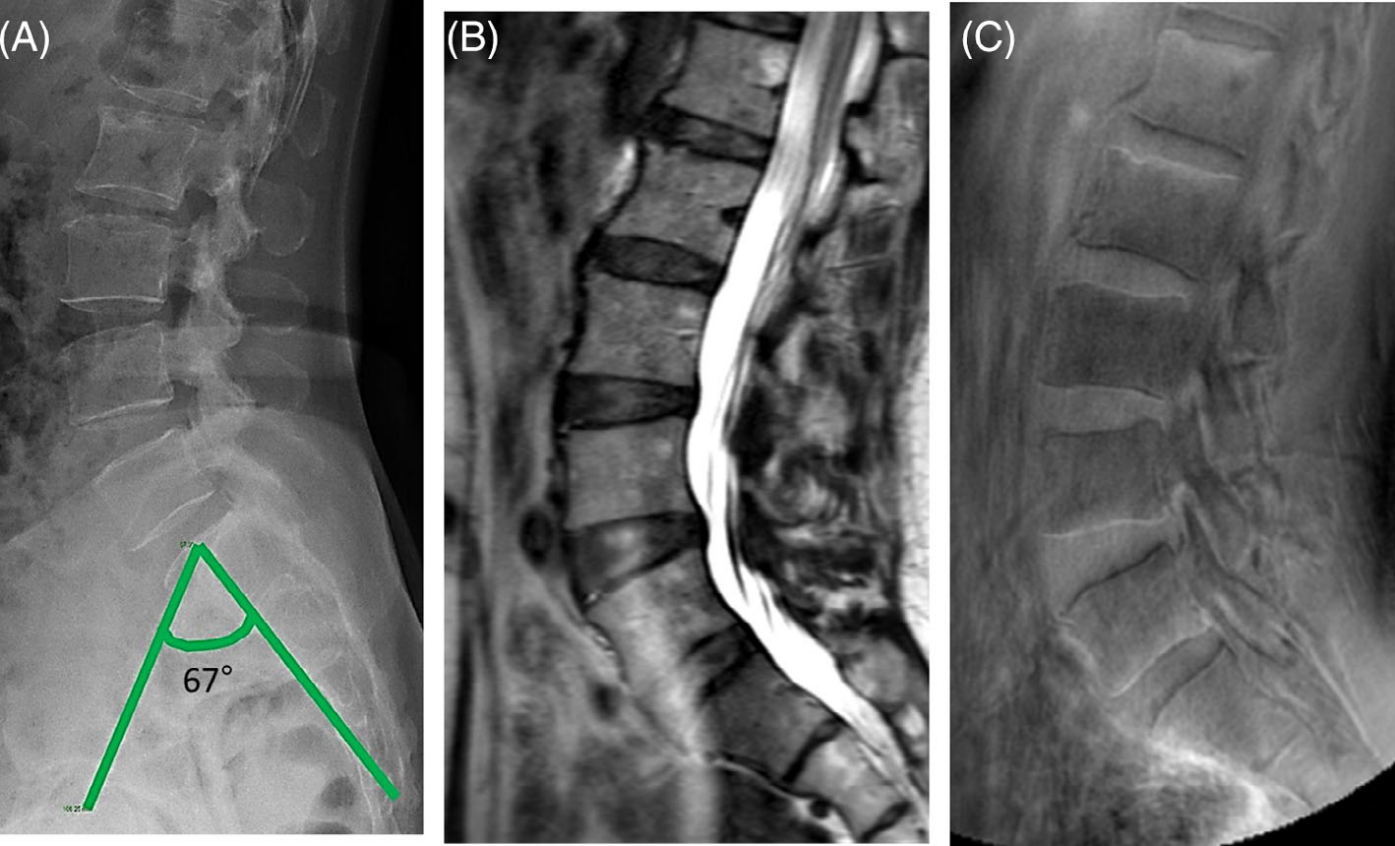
Images depicting the lumbar spine of 53 year‐old female by three different imaging techniques. A, Lateral plain radiograph showing high pelvic incidence of 67°. B, T2‐weighted MRI showing no Modic changes. C, Ultra‐short time‐to‐echo (UTE) with no UTE disc signs
